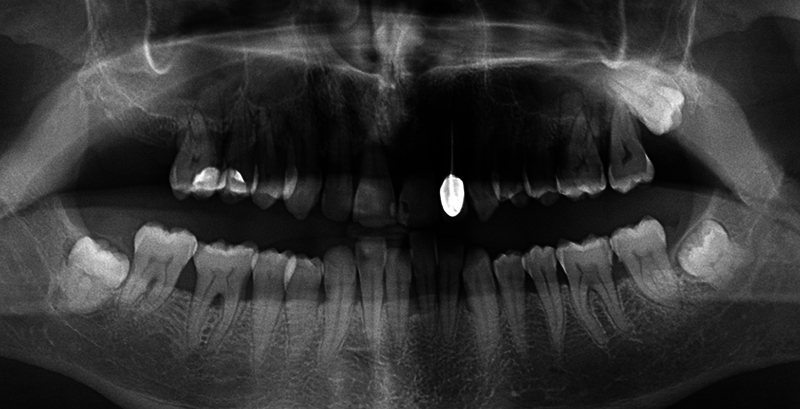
Clinical case no 1-Panoramic X-ray.
